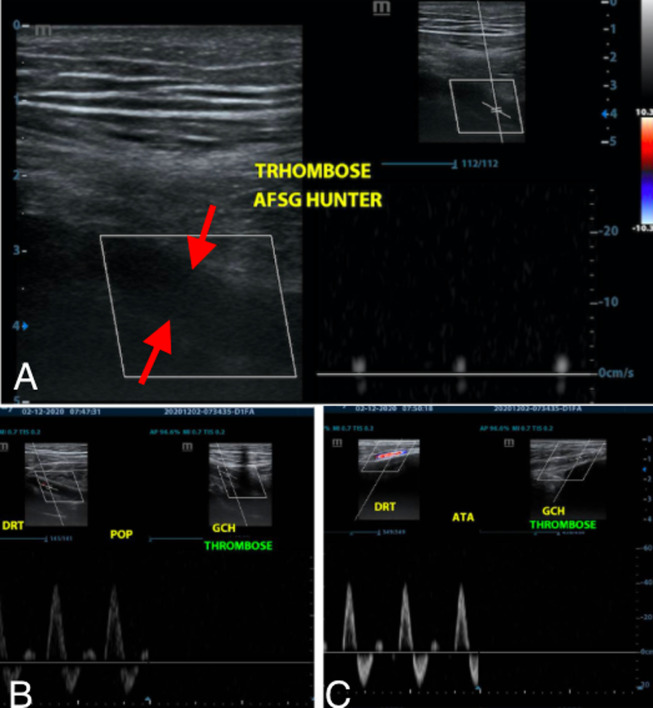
example of a Doppler ultrasound scan of a 30-year-old patient with no known pathological condition, received for pain in the left lower limb for 3 days; the ultrasound doppler allowed the diagnosis of arterial thrombosis of the left lower limb involving the femoral; (A) popliteal; (B) and tibial; (C) with a hypoechogenic occlusive thrombus (delimited by the red arrows); in this patient, despite the absence of symptoms suggestive of COVID-19, the PCR test was positive
